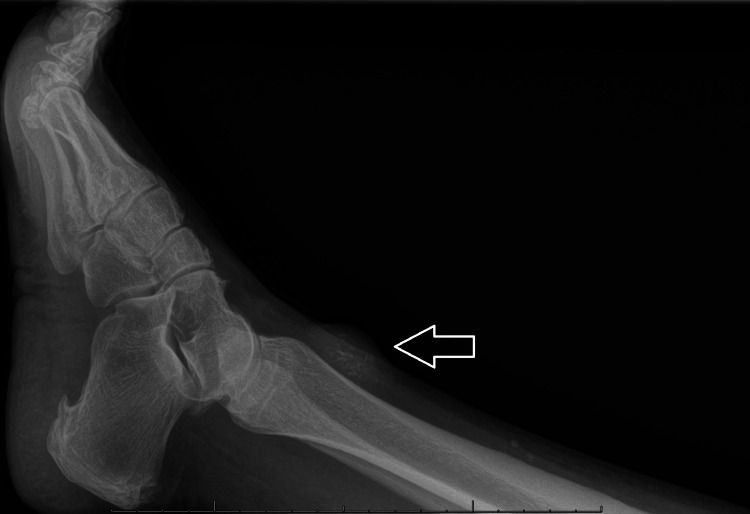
Lateral radiograph of right ankle demonstrating soft tissue mass with calcifications (arrow).
